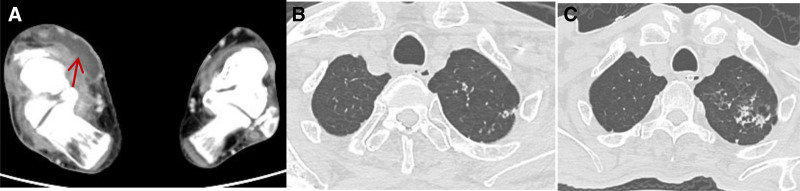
(A) Contrast-enhanced computed tomography (CT) scan of the right ankle indicating swelling of the soft tissue around the right talus (red arrow), (B) thoracic CT showing multiple hyperdense nodular shadows of varying sizes in both lungs, (C) pulmonary lesion progression following anti-tuberculosis treatment. CT = computed tomography.
